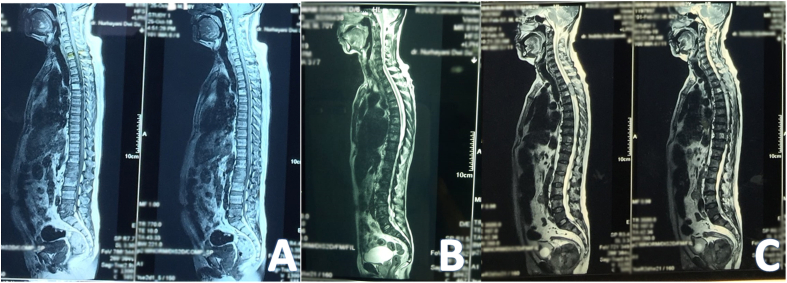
MRI wholespine; A. Case 1 (Mr.M), B. Case 2 (Mr.B), C. Case 3 (Mr.I).
